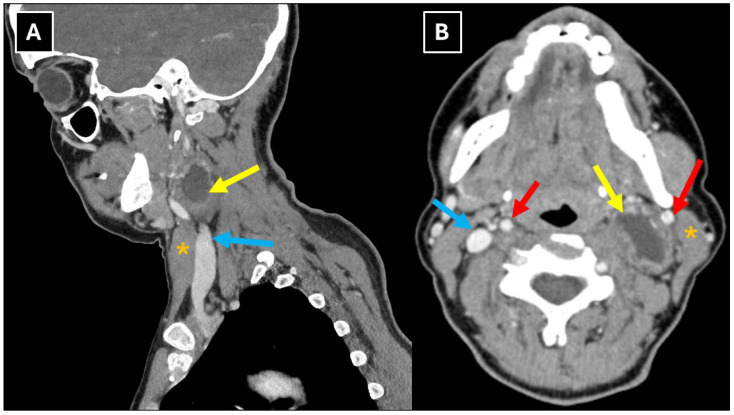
Contrast-enhanced neck CT with soft tissue window in sagittal plane (A) and axial plane (B) shows a left carotid space mass with internal cystic appearance (yellow arrows) and a thick, contrast-enhancing capsule, located medially to the sternocleidomastoid muscle at the C3-C4 level. The internal jugular vein is compressed. Red arrows—internal carotid artery, blue arrows—internal jugular vein, orange asterisks—sternocleidomastoid muscle.
